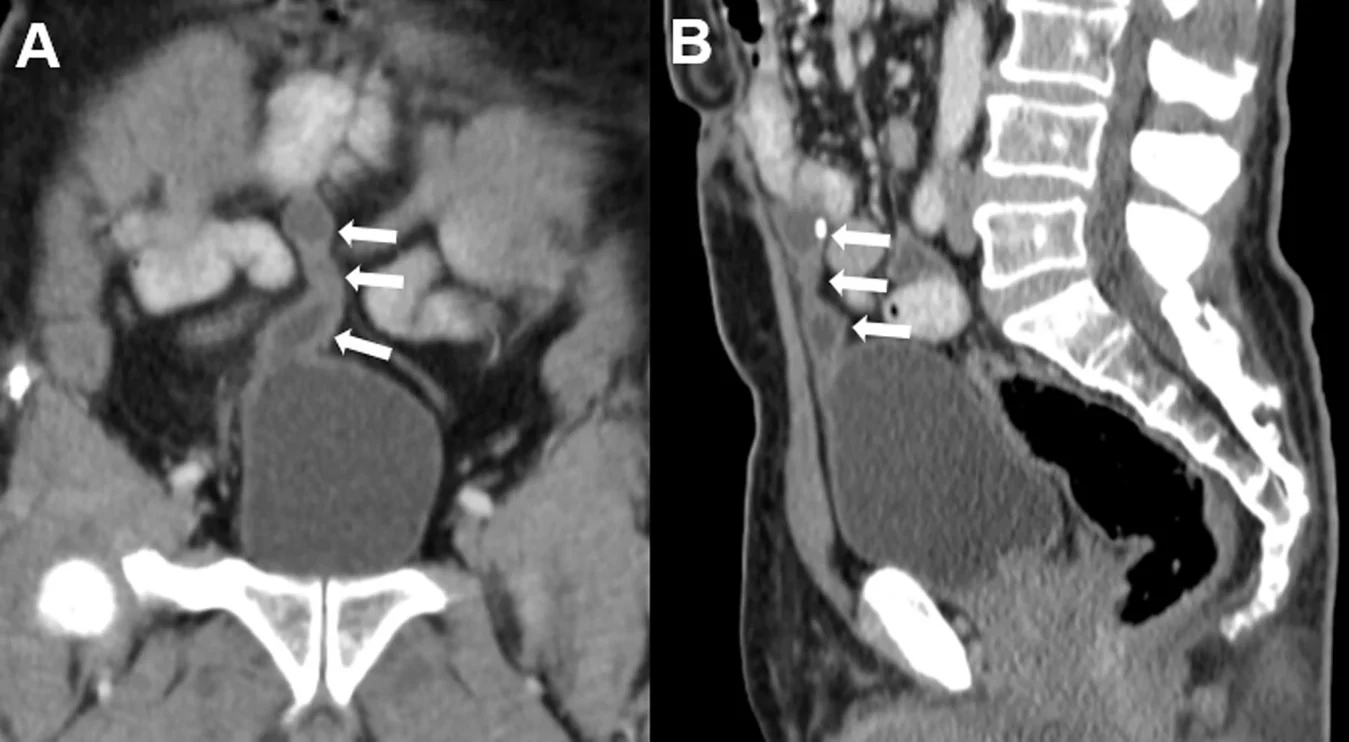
Coronal (A) and sagittal (B) CT images demonstrate a tubular-shaped lesion (arrows) extending from the bladder apex to the umbilicus, consistent with a vesicourachal diverticulum, in a 73-year-old male patient with a history of gastric cancer
